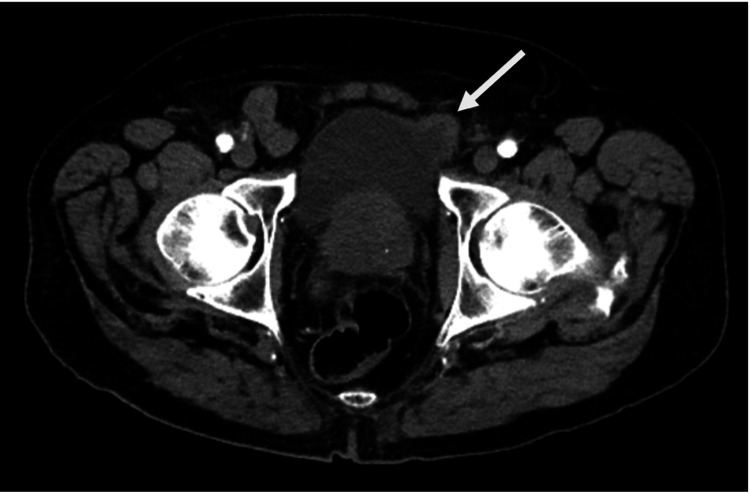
Axial arterial-phase CT scan through the lower pelvis showing a left anterior bladder bulge (white arrow) CT: computed tomography
